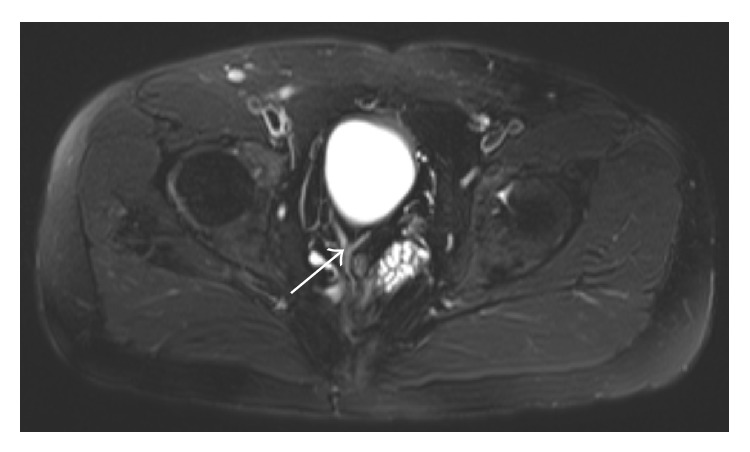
MRI of pelvis at the level of the bladder and rectum. Arrow: colovesicular fistula.
